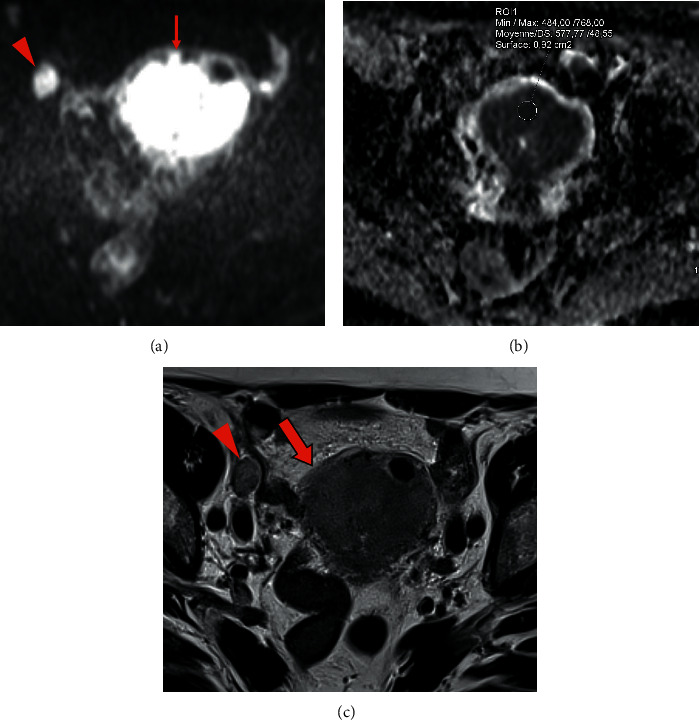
Pelvic MRI of endometrial stromal sarcoma: (a, b, c) axial DWI b1000 showing a large intrauterine tumor with high signal intensity and lobular/irregular tumor contours (arrow) (a) and low ADC value (0.58 × 10^−^³ mm^2^/s) (b), and an obturator lymph node metastasis (arrowhead) (a). Note the intermediate T2 signal intensity of these two lesions and the blurred contours of the tumor (empty arrow) (c).
